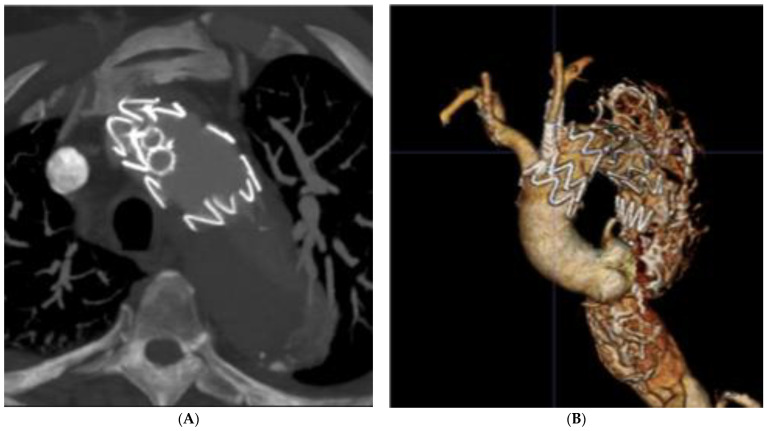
Post-operative CT scan 3D volume rendering (A) of the in situ laser fenestrations of the left common carotid and left subclavian arteries. The 3D reconstruction (B) shows fenestrations position and patency.
